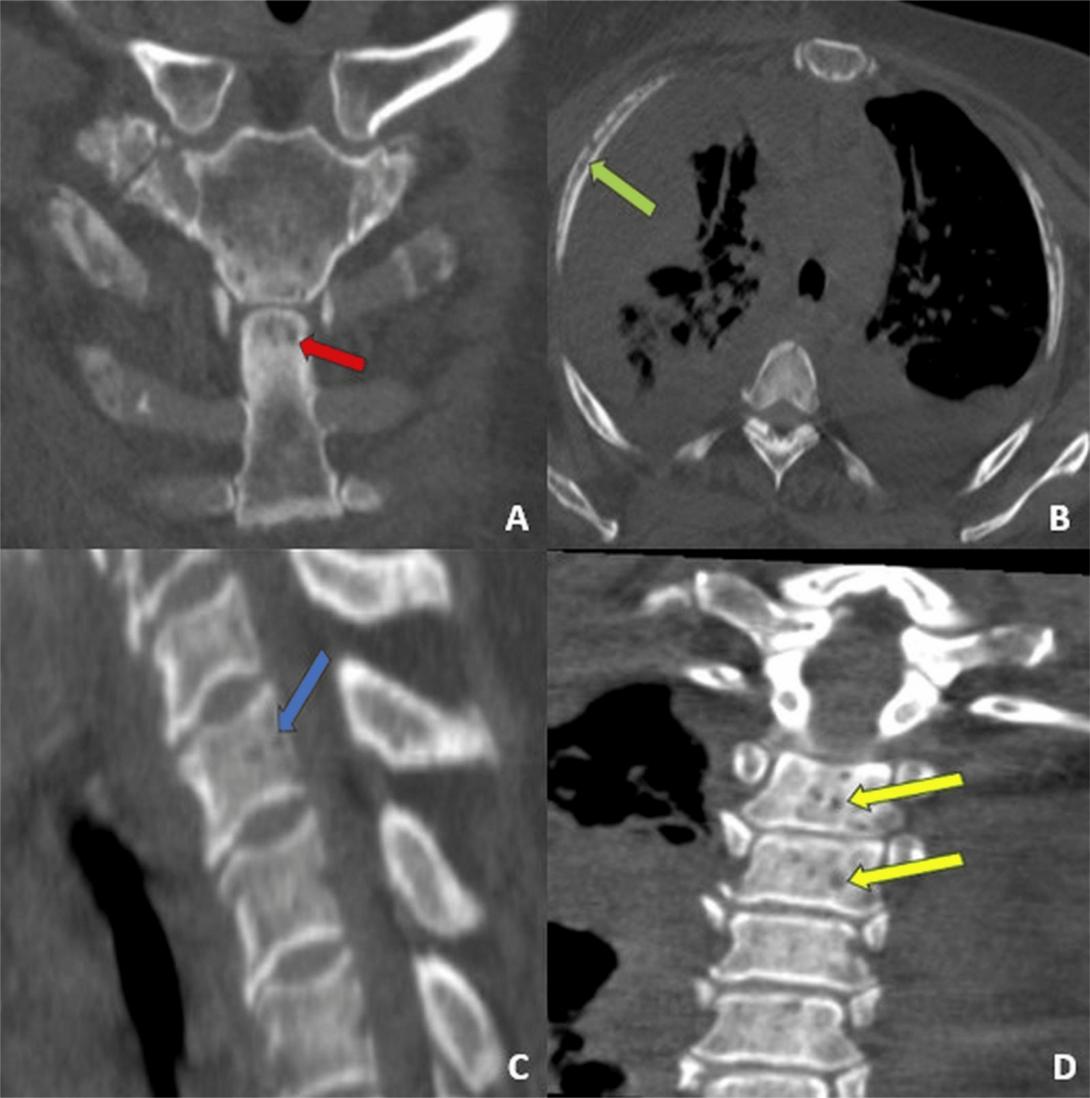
CT findings demonstrating multifocal osteolytic disease (A) Coronal CT image showing a lytic lesion of the sternum (red arrow); (B) Axial chest CT demonstrating an osteolytic lesion of the rib, suggestive of malignant involvement (green arrow); (C) Sagittal reconstruction revealing a vertebral osteolytic lesion with endplate erosion (blue arrow); (D) Coronal CT view highlighting multilevel vertebral body involvement with marked cortical disruption (yellow arrows)
